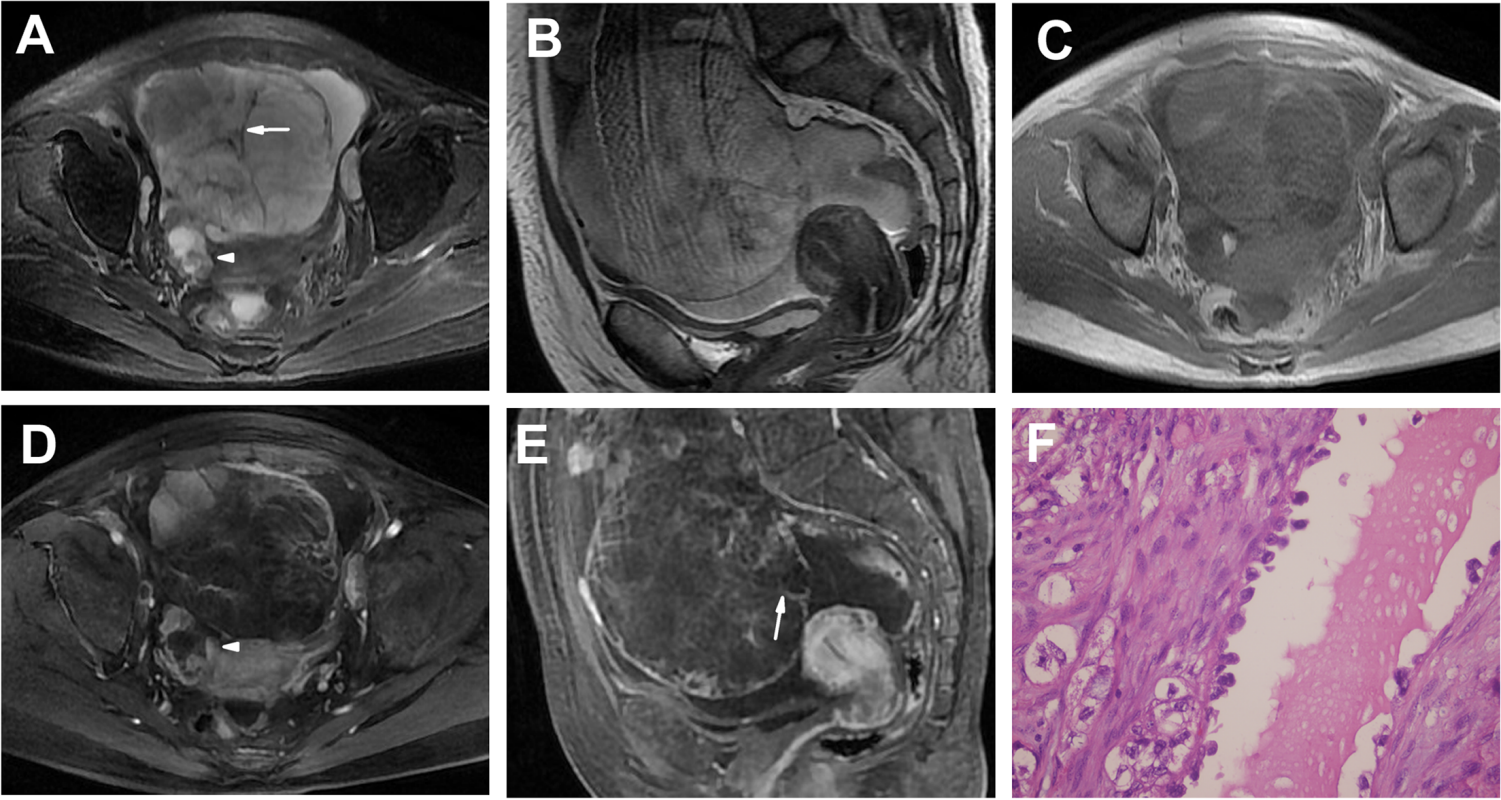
Bilateral OCCCs in a 47-year-old woman (Patient 10 in Tables 1 and 2) presenting with abdominal distension for 2 months. A-C Axial (A), sagittal T2WI (B) and plain (non-contrast) T1WI (C) showing a left-side, large, well-defined multilocular cystic mass with a few irregular lumen solid protrusions and many irregular septations (> 3 mm, arrow) and similar MRI findings of a right-side, small, multilocular cystic mass with protrusions (arrow head). The SI of the cyst was high on T2WIs and low on T1WI. The solid protrusions had heterogeneous iso-slightly high SI on T2WIs and iso-SI on T1WI. Bulk ascites were detected. D, E Enhanced T1WIs showing markedly heterogeneous and prolonged enhancement solid protrusions and septations (arrow). F The tumor shows a cyst lined with hobnail cells. Secretions were found in the lumen of the cyst. (HE 40 & 10).
